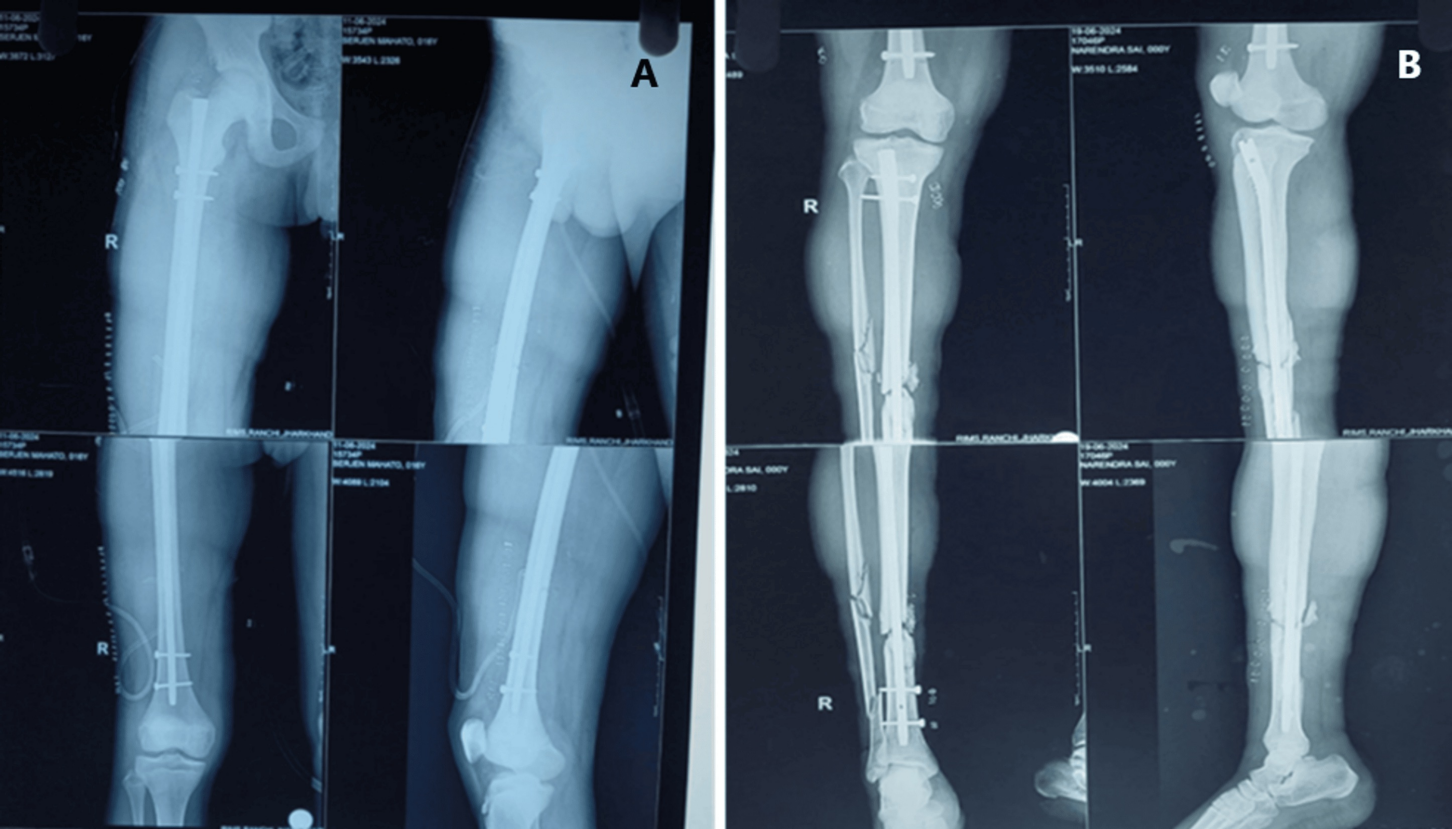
Postoperative radiograph showing internal fixation with nailing in type I floating knee injury: (A) mid-shaft femur fracture managed with interlocking nail and (B) mid-shaft tibia fracture managed with interlocking nail
